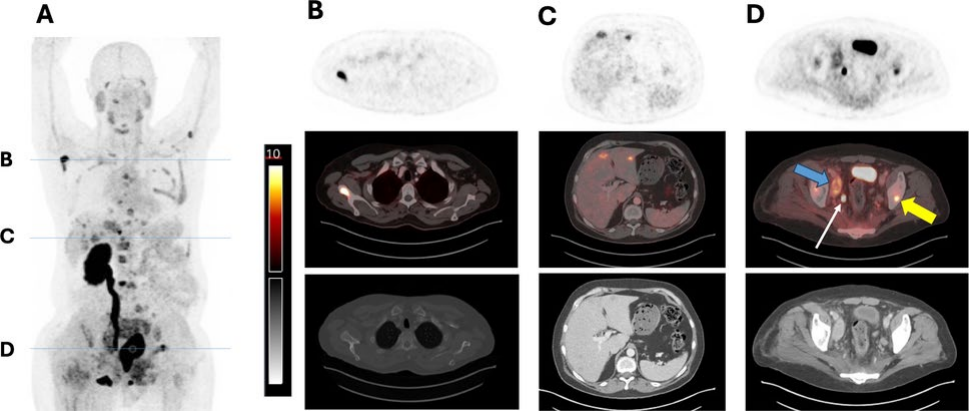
Single-patient compassionate use of [^68^ Ga]Ga-PSMA-1-DOTA. A Compassionate use of [^68^ Ga]Ga-PSMA-1-DOTA in a PET scan of a patient with metastatic prostate cancer who had progressed after radioligand therapy with ^177^Lu-PSMA-I&T, maximum intensity project (MIP). The patient is status post left nephrectomy for benign disease. Note the low uptake in the salivary glands, liver and spleen compared to PSMA-avid metastases. B-D Selected trans-axial cross-sections cut from panel A showing from the top: PET, PET/CT overlay, and CT at B metastasis in the right scapula, not osteoblastic on CT; C lower liver uptake and 2 liver metastases; D right external iliac metastasis (blue arrow) and left iliac metastases (yellow arrow) and ureter (thin arrow). The tumor to salivary uptake ratio (t/s) was: t/s = 5.63 for B; t/s = 2.08 for C; and t/s = 2.58 (blue arrow) and 2.84 (yellow arrow) for D. There are several other metastases throughout the body whose cross-sections are not shown
